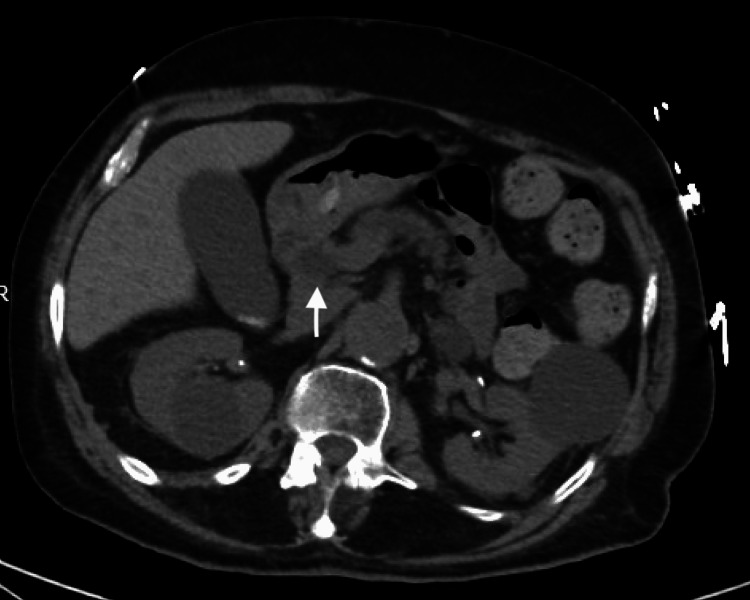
CT Abdomen without contrast showing dilated pancreatic duct (white arrow)
